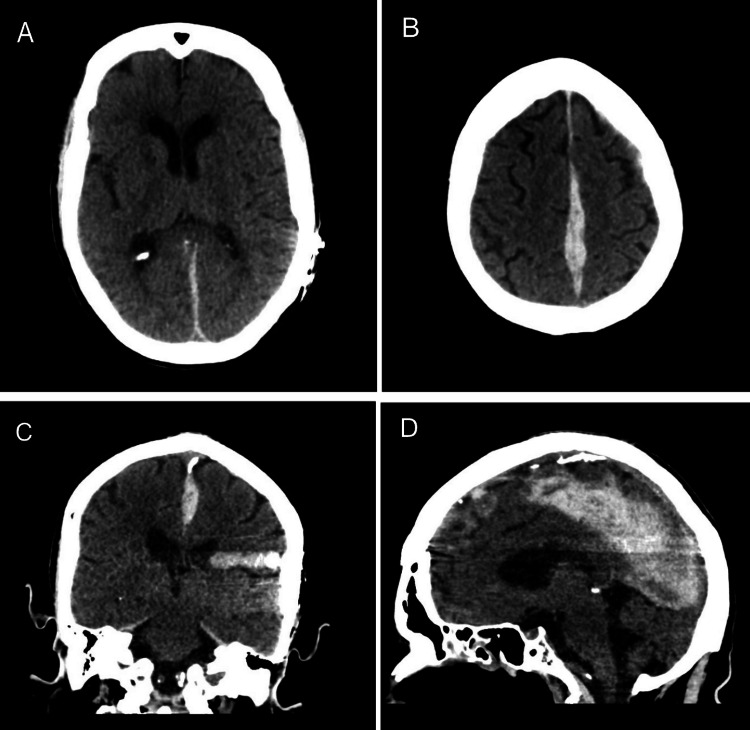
Non-contrast CT scan of the head. Panels A and B show the axial views of a hyperattenuating parafalcine subdural hematoma measuring up to 11 mm in thickness. Panel C shows a coronal view of the hematoma in the location of the falx cerebri, with some mild artifacts on the right, secondary to the patient's cochlear implant. Panel D shows a sagittal view of the parafalcine subdural hematoma extending into the falx cerebelli.
